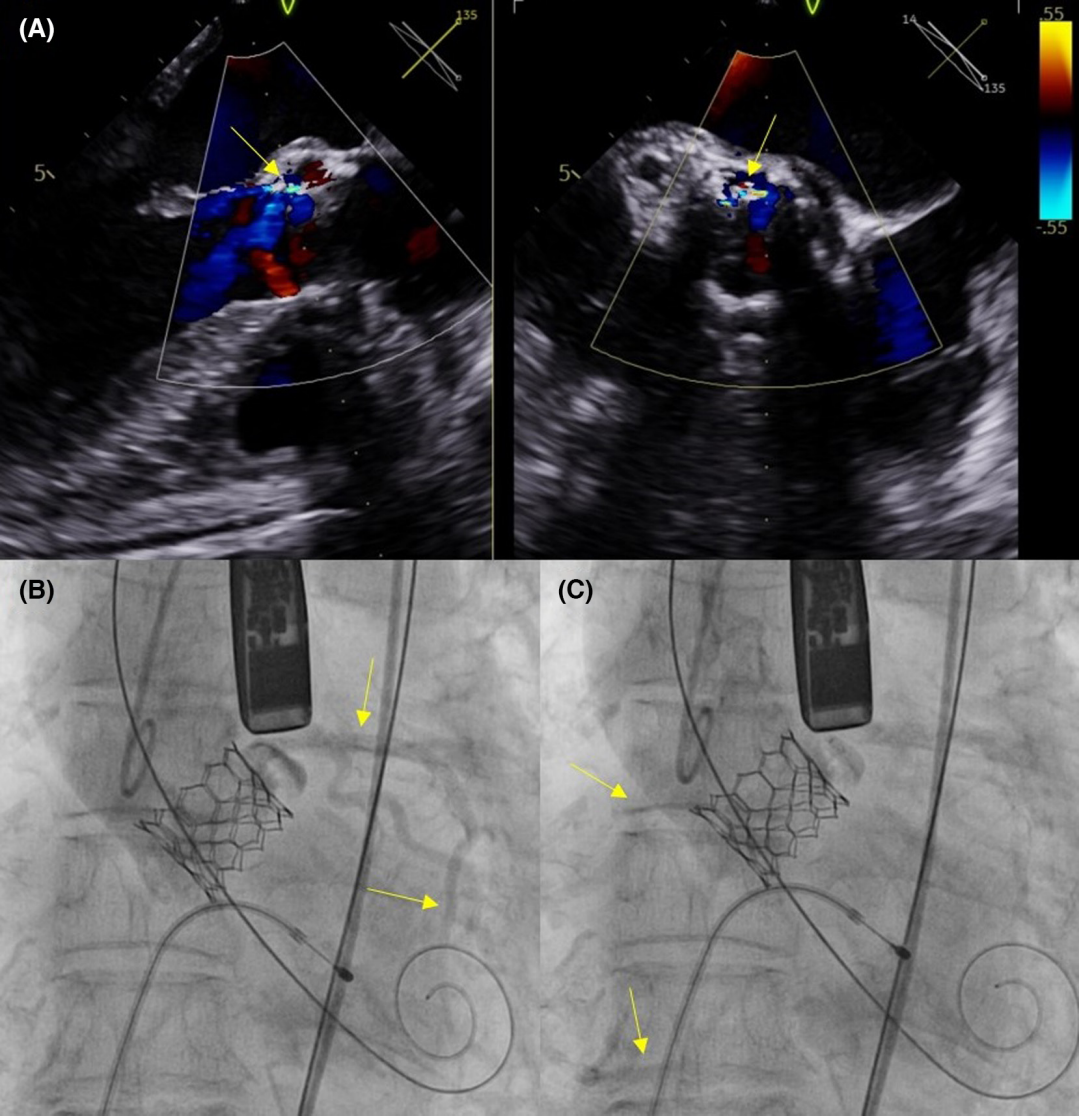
Post TAVI procedural findings (A) Transesophageal echocardiography showed minimal paravalvular leakage (arrow). (B, C) Coronary artery blood flow was intact on aortography after the TAVI procedure.
